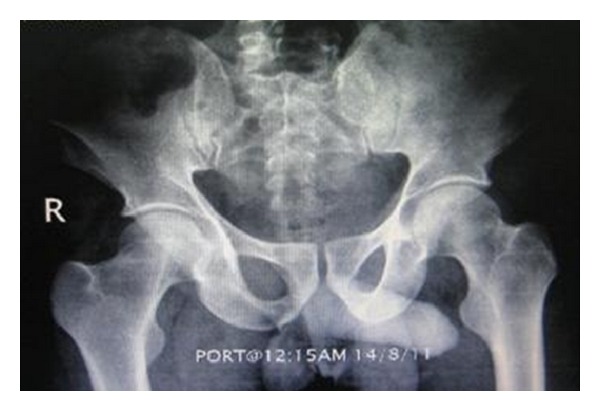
Pelvic X-ray of the patient showing no fracture.
